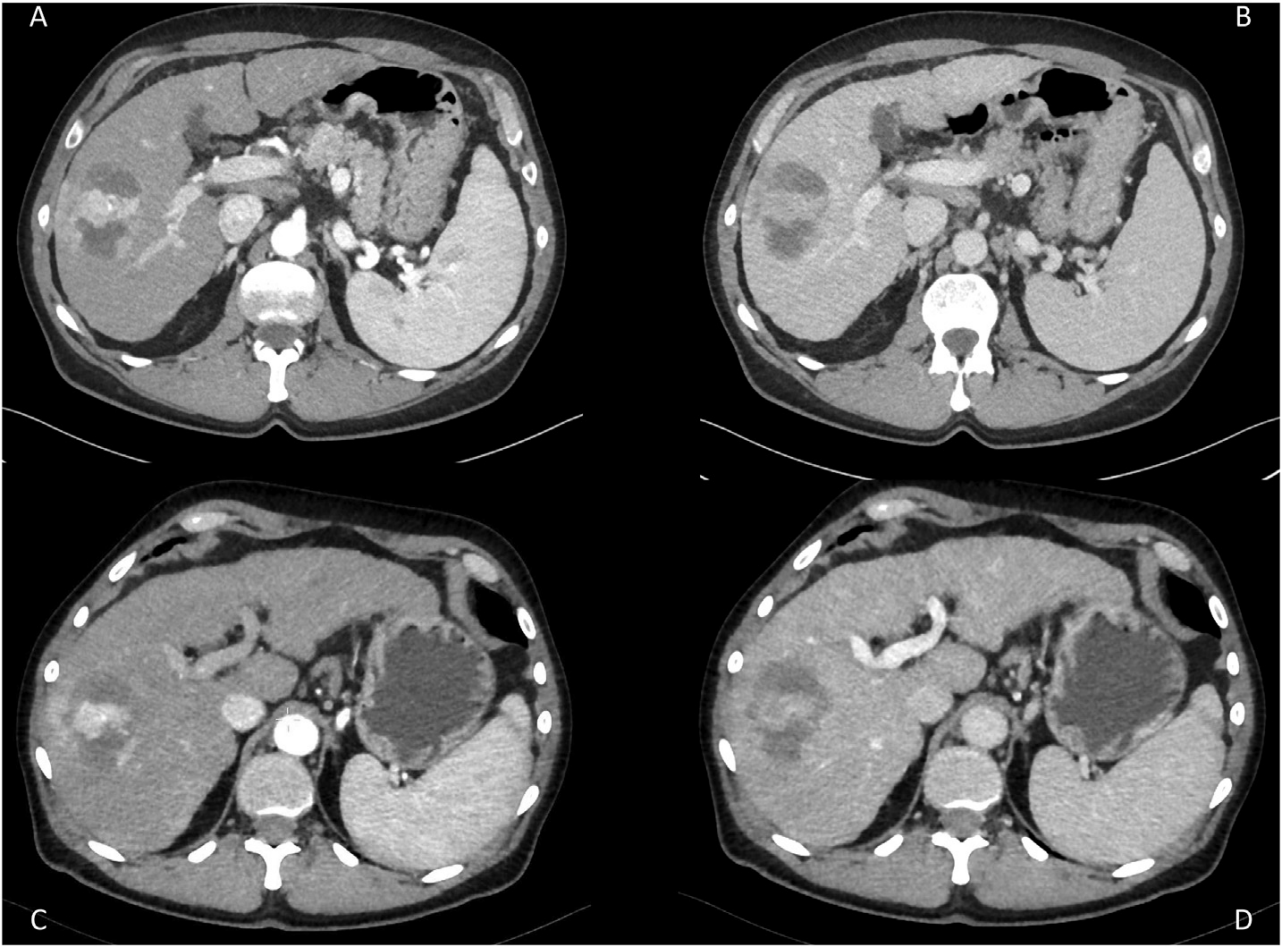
Contrast-enhanced cross-sectional images of abdominal 4-phase CT with A) arterial and B) PV phase and reconstructed images from CTP with C) A-CTP and D) PV-CTP phases.
